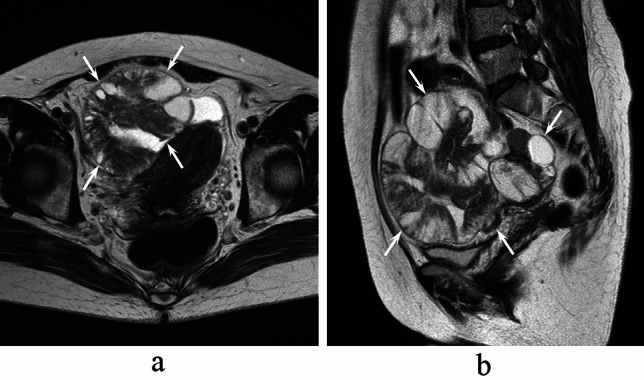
A 50-year-old woman with right OMCC. Axial (a) and sagittal (b) T2-weighted images show a multilocular cystic mass with the mille-feuille sign (arrows)
